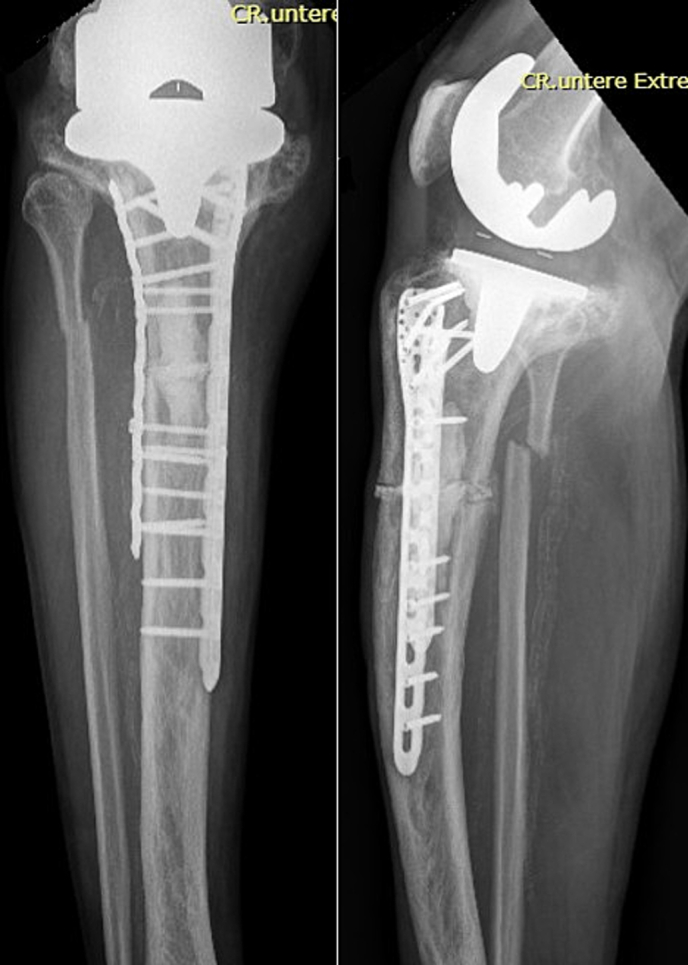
Six-week postoperative AP and lateral radiographs of the right knee and lower leg demonstrate early signs of fracture consolidation without signs of hardware loosening.
